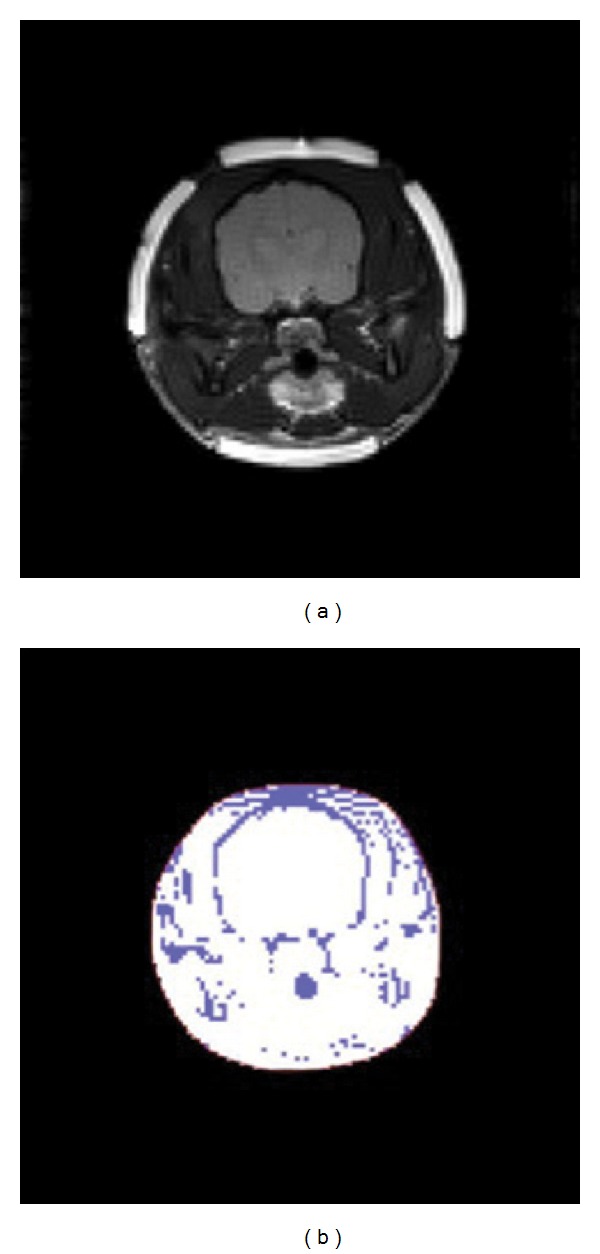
Defected region select using threshold (a) MR image and (b) defected region by the thresholding (purple color).
